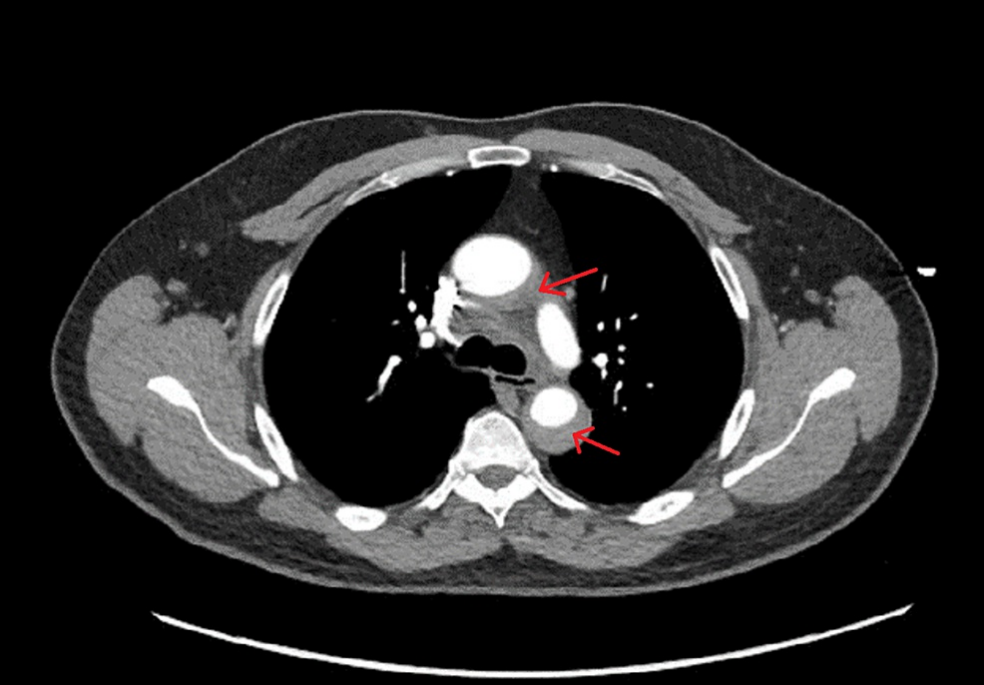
CT imaging showing an axial view of the intramural hematoma surrounding the lumen of the aorta (red arrows).
